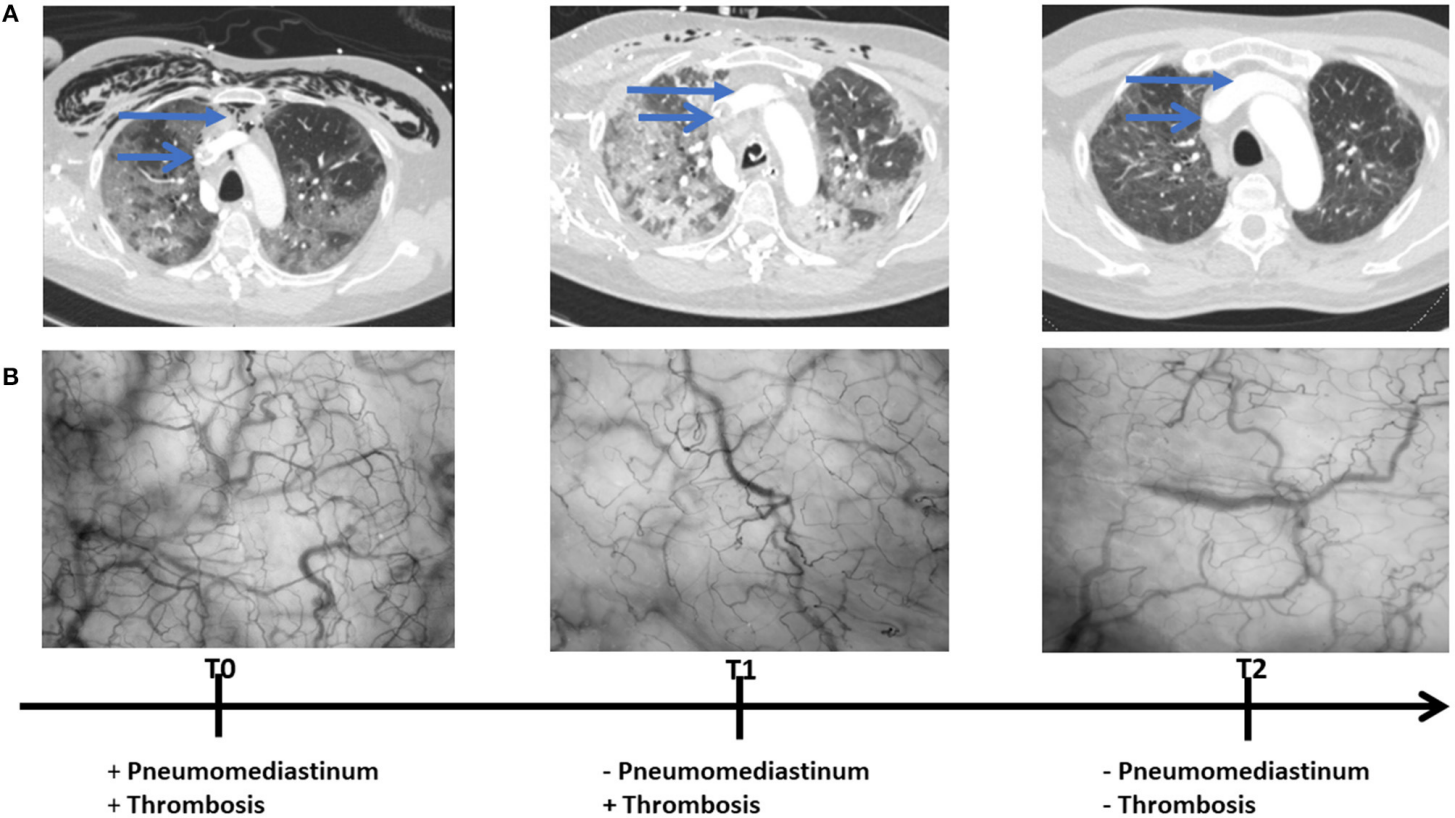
Chest CT (A) and microcirculatory images (B) obtained at 5th (T_0_), 19th (T_1_), and 25th (T_2_) day of intensive care admission.
